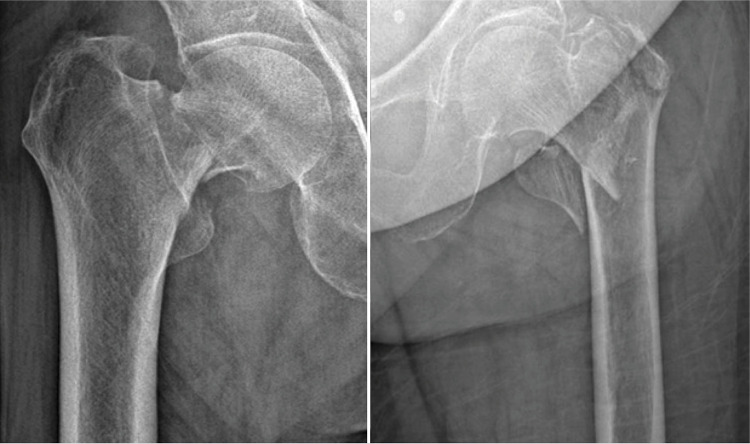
Radiographs of a 78-year-old male patient with a displaced femoral neck fracture and an 83-year-old female patient with an unstable intertrochanteric fracture.
